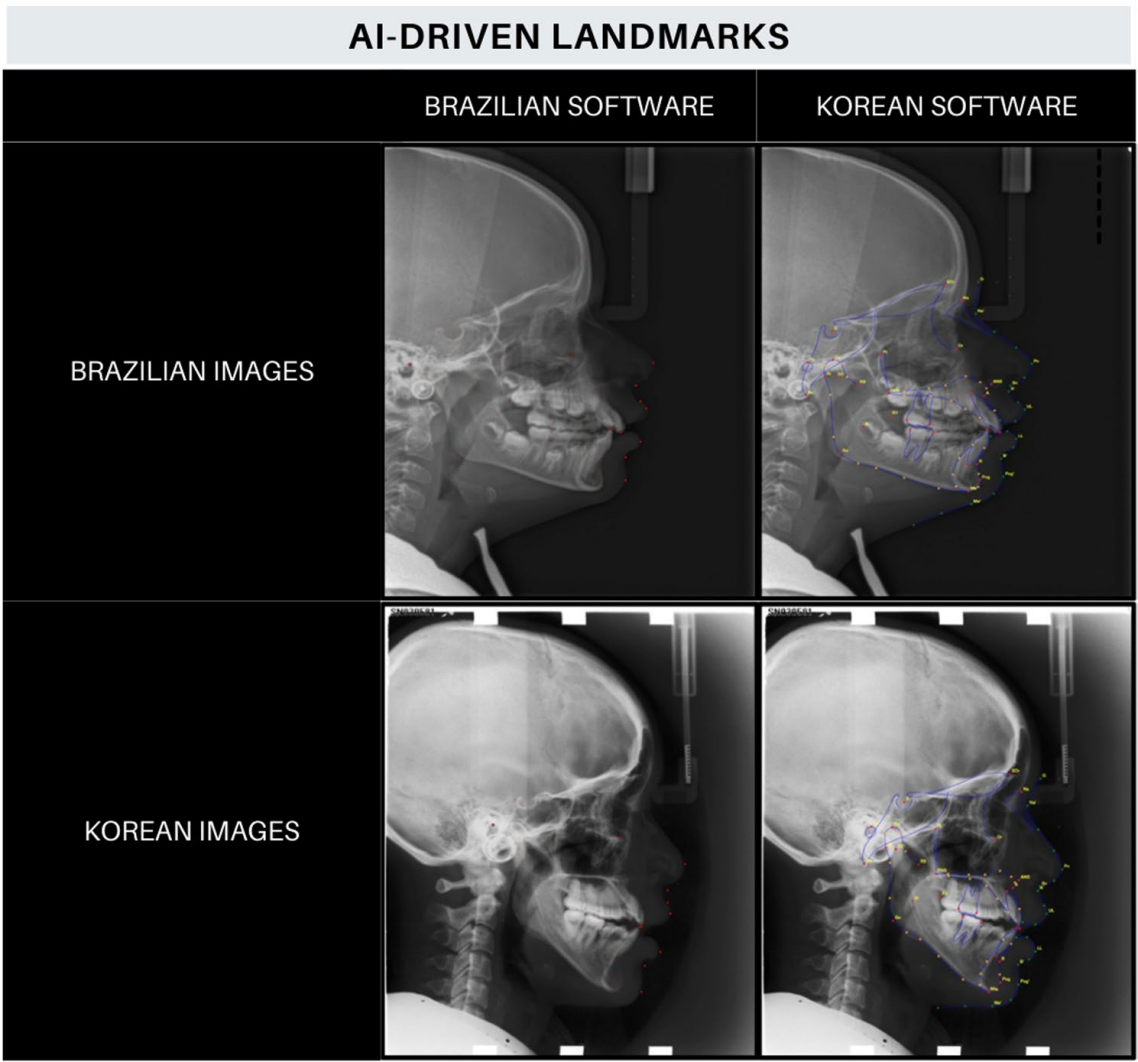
Lateral cephalometric radiographs from the sample images of Brazilian and Korean individuals after automated cephalometric identification performed by the AI-driven software programs CefBot™ and WebCeph™
